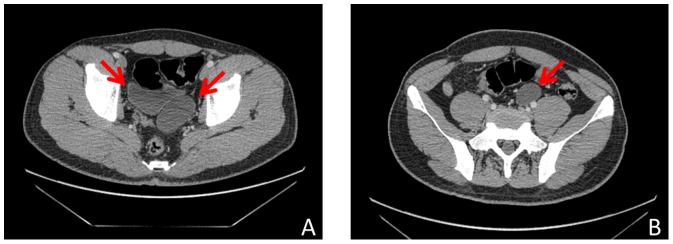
Preoperative CT scan of two patients. (A) The megaureter and bladder could be identified by CT scan. (B) The megaureter could be identified by CT scan.
